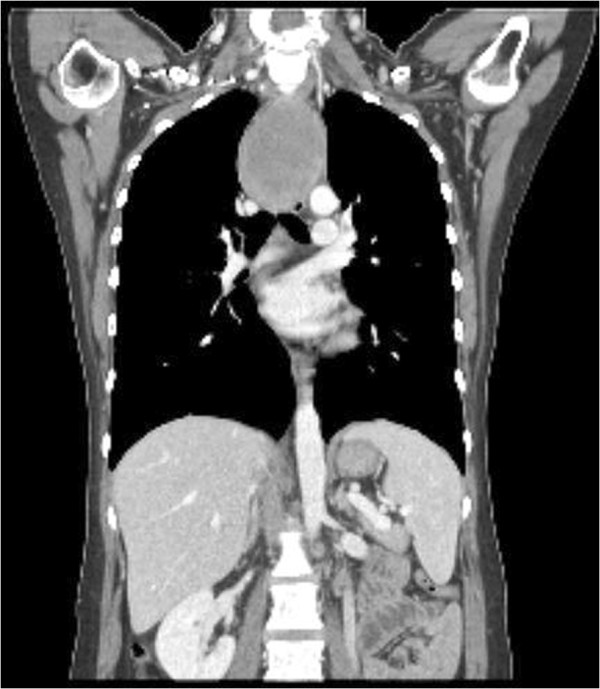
Coronal view of chest CT showed huge mass in the upper thoracic esophagus.
